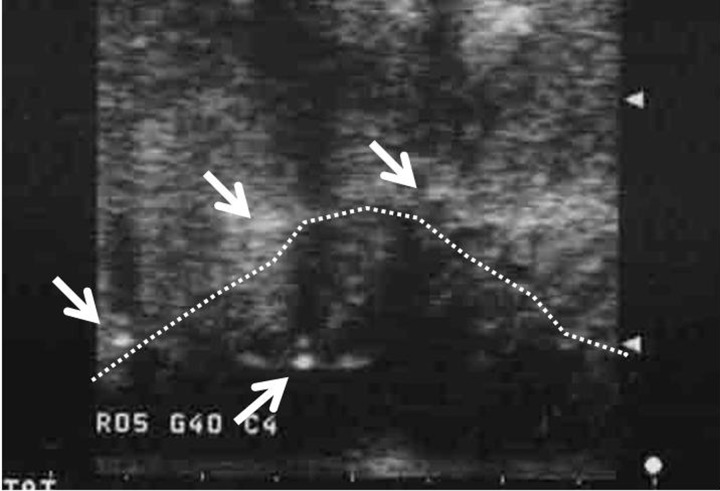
An intraoral ultrasonography image during applicator implantation is shown. Applicators with metal obturators (arrow) and the tumor (dotted line) are clearly visualized.
